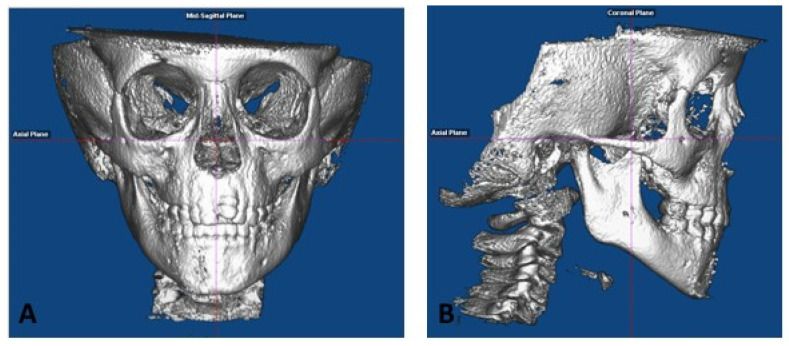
CBCT Orientation: (A) roll and yaw, and (B) pitch, as previously published in [25].
